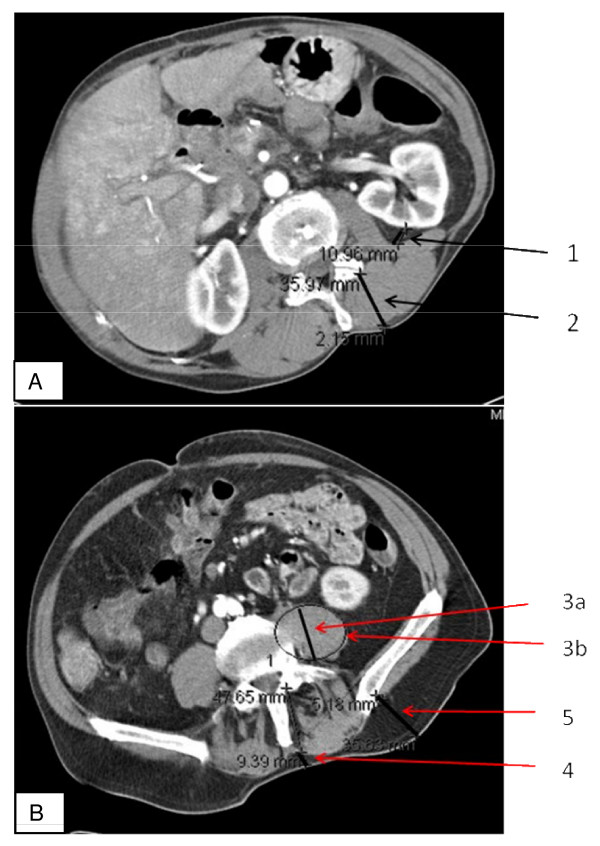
A: CT slide of renal pelvis. [1] perirenal fat: orthographic to renal capsule and to line 2. [2] musculus erector spinae: thickness of the muscle in a parallel line to the dorsal process Figure 1B: CT slide of iliac crest. [3a] thickness of the musculus psoas in a parallel line to the dorsal process. [3b] area of the psoas muscle. [4] subcutaneous fat medial: parallel to the dorsal process. [5] subcutaneous fat lateral: parallel to the dorsal process starting at iliac spine posterior superior area of the psoas muscle.
